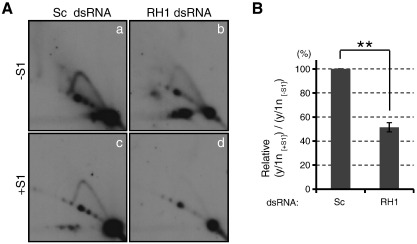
RNase H1 depletion causes enhanced sensitivity to S1 nuclease of mtDNA replication intermediates. (A) Analysis of a *Dra*I-digested mtDNA fragment as in Fig. 2A from cells treated with scramble (Sc) dsRNA or RNase H1-specific (RH1) dsRNA (− S1) (a and b) and those with S1 nuclease treatment (+ S1) (c and d). The images were produced from a single membrane exposed to an X-ray film. (B) Numerical presentation of the Y arc stability against S1 nuclease upon knockdown with RH1 dsRNA. The presentation of the graph is the same as in Fig. 2B. Data represent the mean of 3 independent transfection experiments ± S.E.M. One of the three transfection experiments in Fig. 3B was not used for the production of this graph. Thus the 2D-AGE image from an additional transfection experiment was used instead.  (*p* < 0.01).
